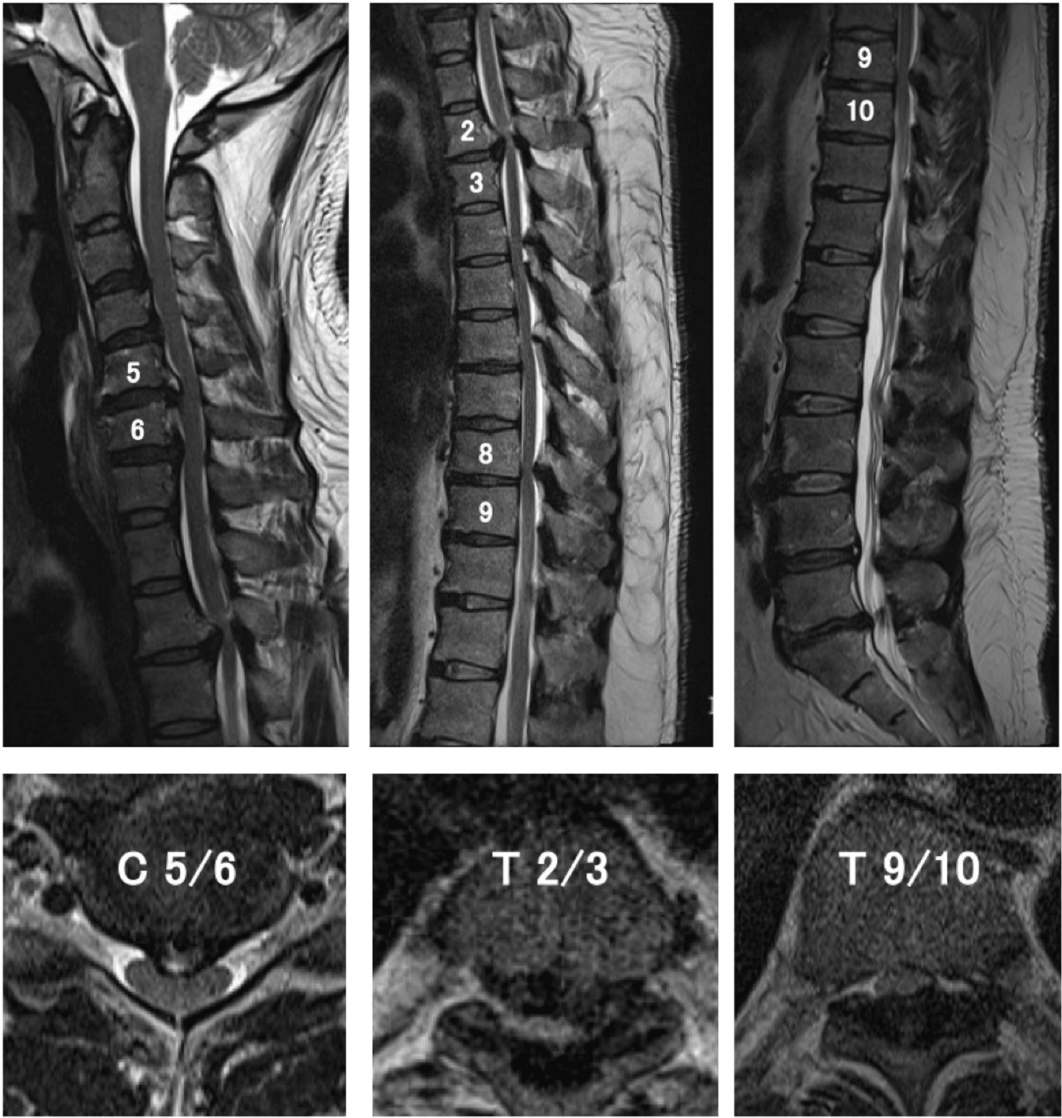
Sagittal and axial T2-weighted imaging showed spinal canal stenosis caused by ossification of the posterior longitudinal ligament and ligamentum flavum extending from the C3/4 to the C6/7 interspaces and at the T2/3 and T9/10 levels. Spinal cord compression and signal change in the spinal cord are visualized at T2/3.
